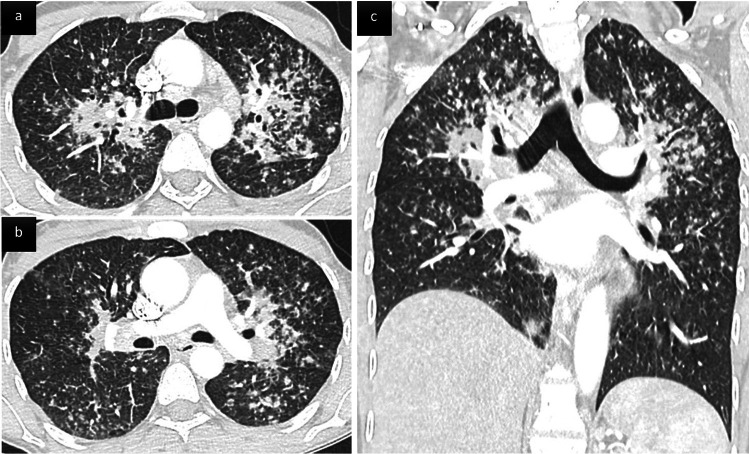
Sarcoid-like infiltrative lung disease following systemic aspergillosis in a 46 yo patient with chronic granulomatous disease (CGD). Axial (a, b) and coronal (c) CT scan images show innumerable small nodules with a peri-lymphatic distribution (peri-broncho-vascular, centrilobular and subpleural) predominant in the upper parts of the lungs, associated with perihilar confluence of nodules, and enlarged hilar and mediastinal lymph nodes. Granulomas were confirmed on transbronchial biopsies
